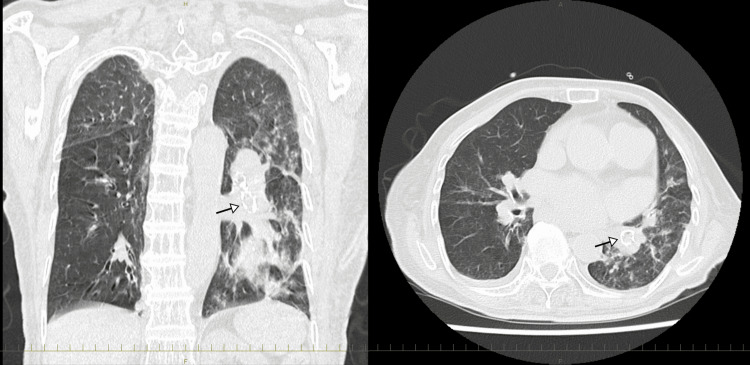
The CT image showing sputum retention in the left lower lobar bronchus Aspiration-related hypoxemia and choking led to sputum retention (arrow), which triggered the need for endotracheal intubation and mechanical ventilation.
